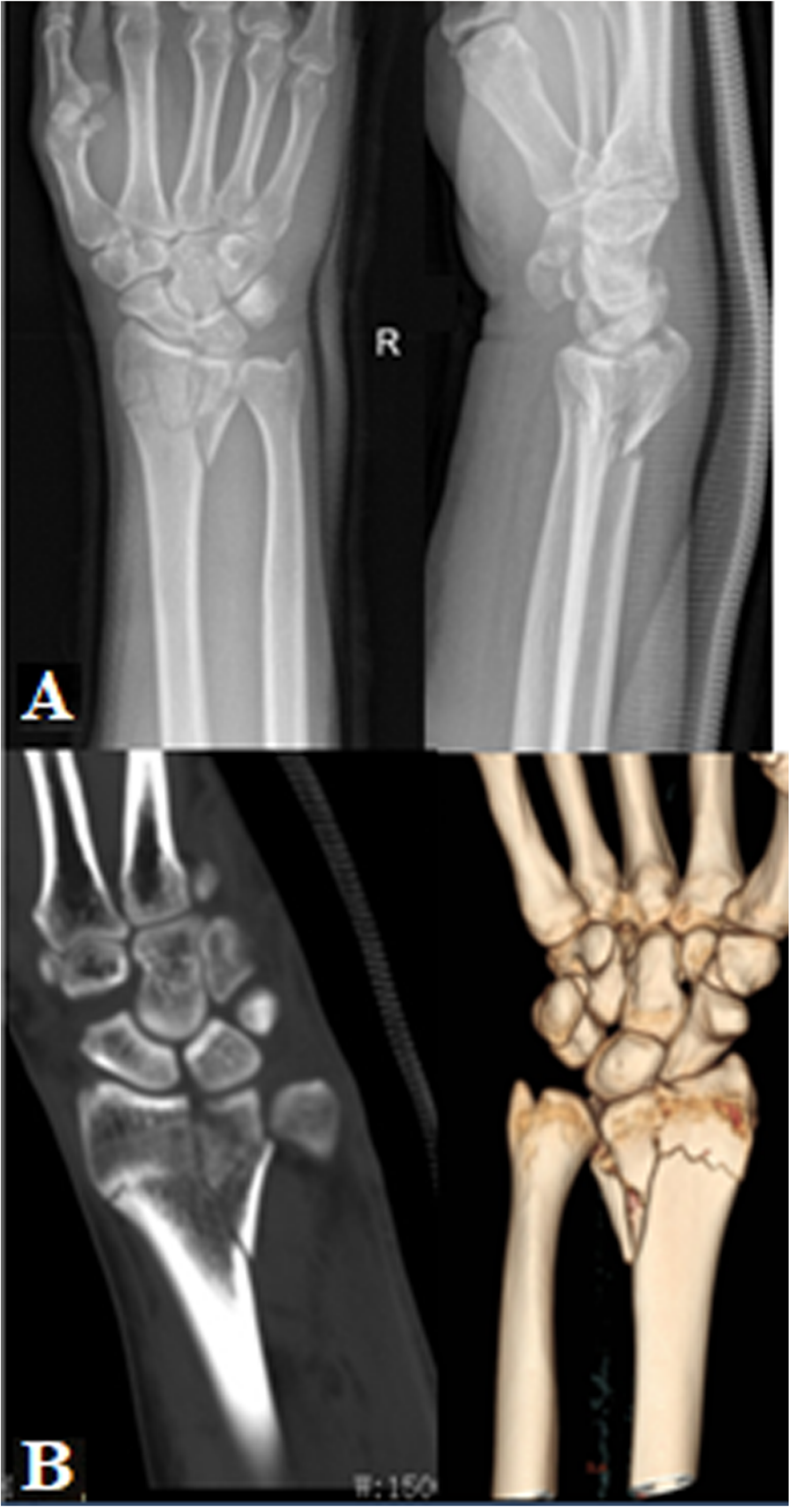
PA X-ray (a) and CT scan (b) of the metaphyseal radius column fracture
